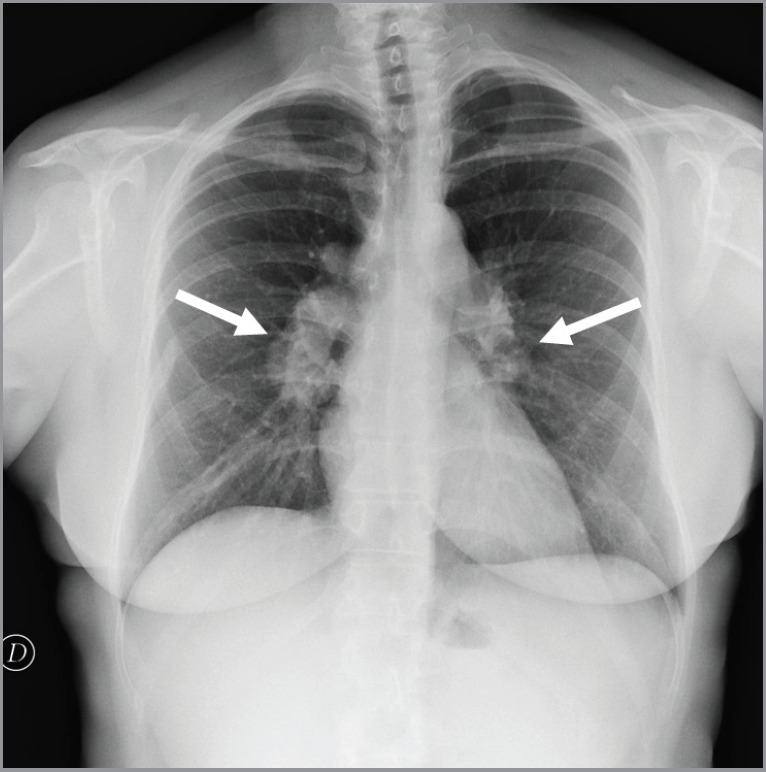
Chest x-ray showed bilateral hilar lymphadenopathies (arrows) suggestive for chronic granulomatous disease.
